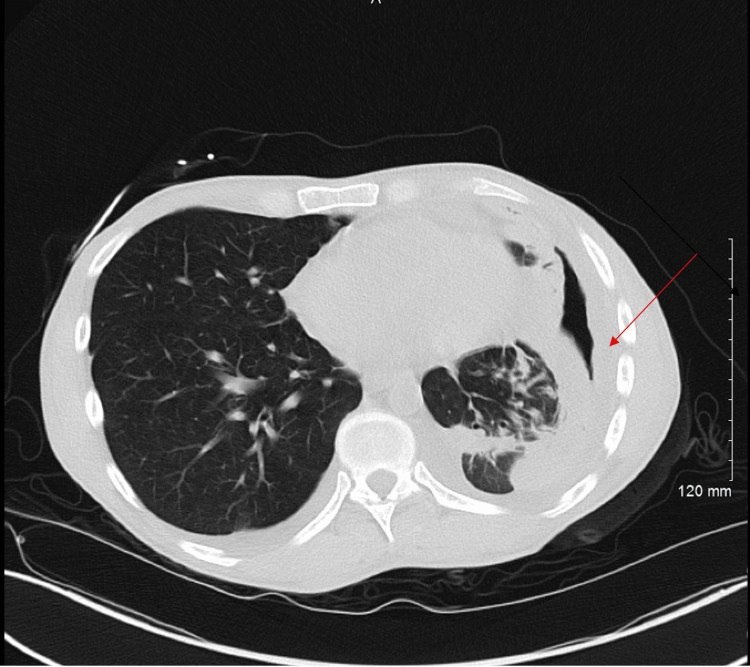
CT of the chest (axial) Axial CT of the chest shows hydropneumothorax on the left, with thickening of the visceral pleura (red arrow) is observed.
